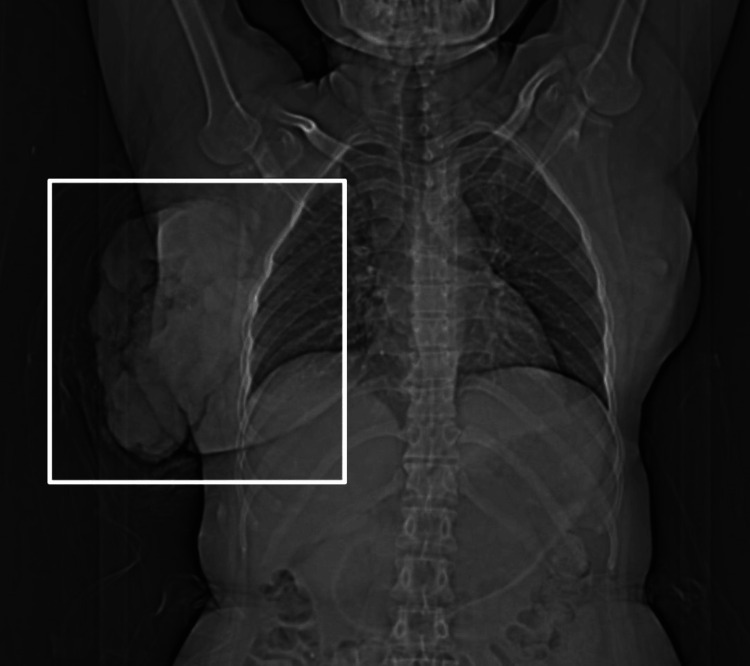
Chest radiograph depicting the growth over the right breast
